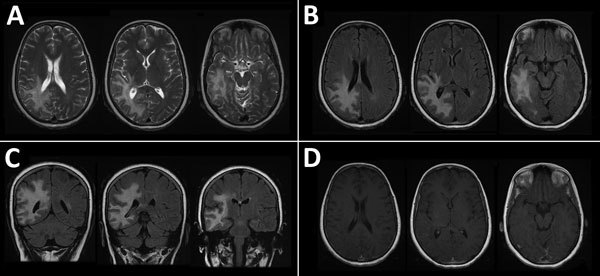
Magnetic resonance imaging of the brain of a 46-year-old immunocompromised woman with central nervous system brucellosis granuloma and white matter disease, Saudi Arabia. A) Axial T2 images showing hyperintensity in the right frontoparietal lobe and right temporal lobe. B) Axial fluid-attenuated inversion recovery (FLAIR) and C) coronal FLAIR images showing that hypersensitivity extends to U-fibers without involvement of the cortex. D) Gadolinium-enhanced image showing that no appreciable mass effect and no central or peripheral enhancement after administration of gadolinium were observed. Each image within each panel shows involvement in different levels of frontal, parietal, and temporal lobes.
